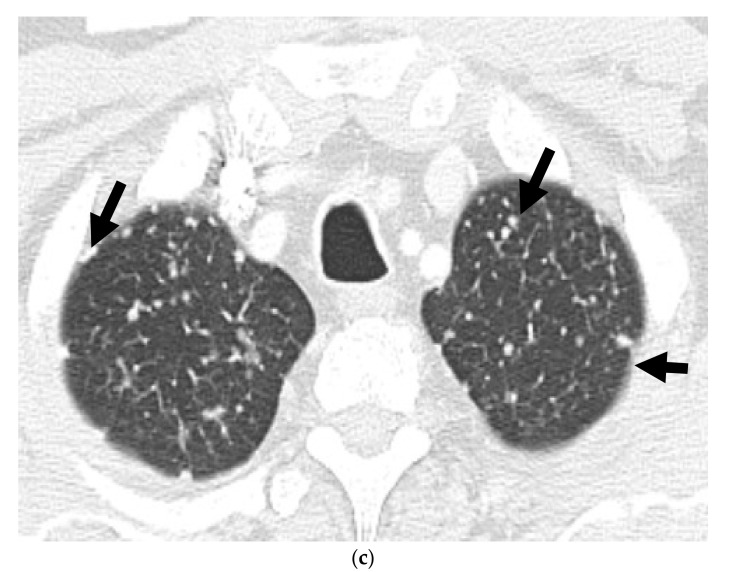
A 68-year-old man with palpable prostatic mass on rectal examination. Serum PSA was 0.3 ng/mL. Axial FDG-PET (a) and corresponding anatomic CT (b) of the PET-CT shows intensely hypermetabolic prostatic mass (long arrow) and pelvic bone metastases (short arrows). Axial lung window reformat of the CT chest (c) shows numerous small pulmonary nodules suspicious for metastases (arrows). Prostate biopsy showed high-grade carcinoma with neuroendocrine differentiation.
